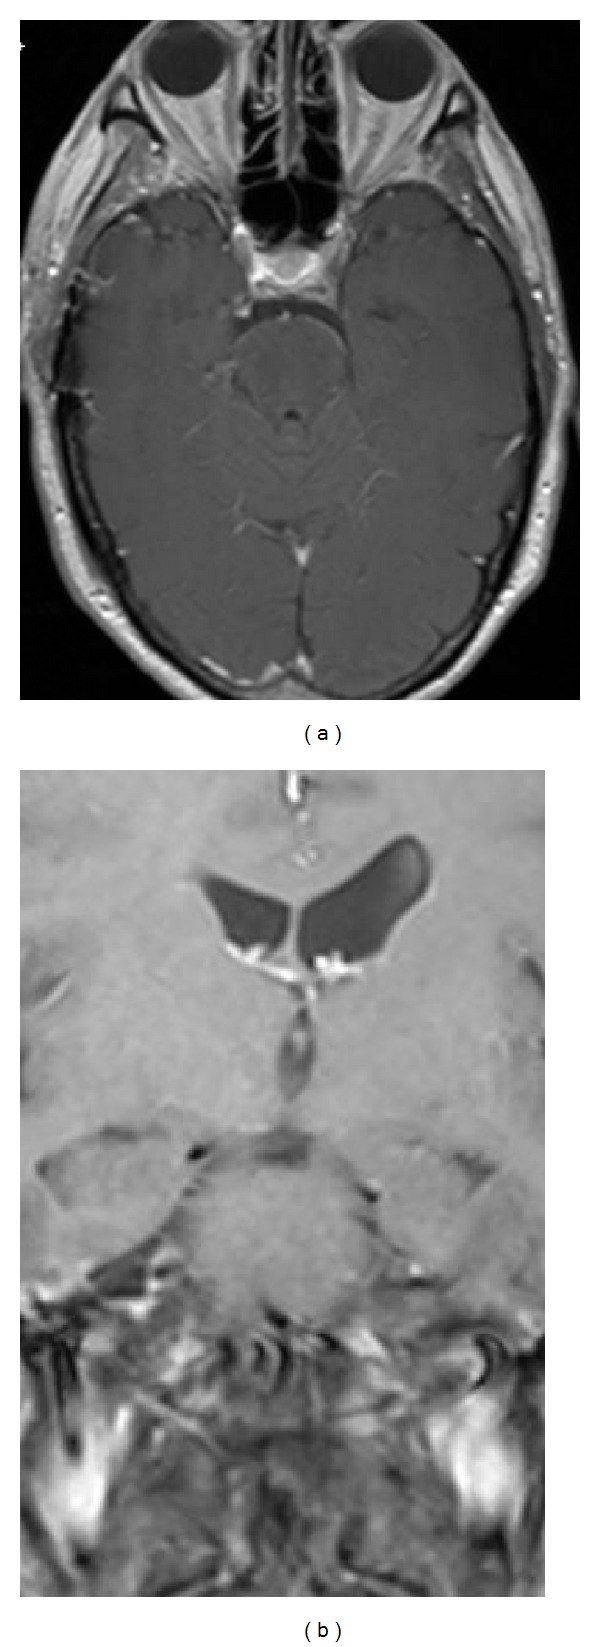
Postoperative MRI brain with contrast axial (a) and coronal (b) T1-weighted images reveals complete resection of the trochlear schwannoma.
